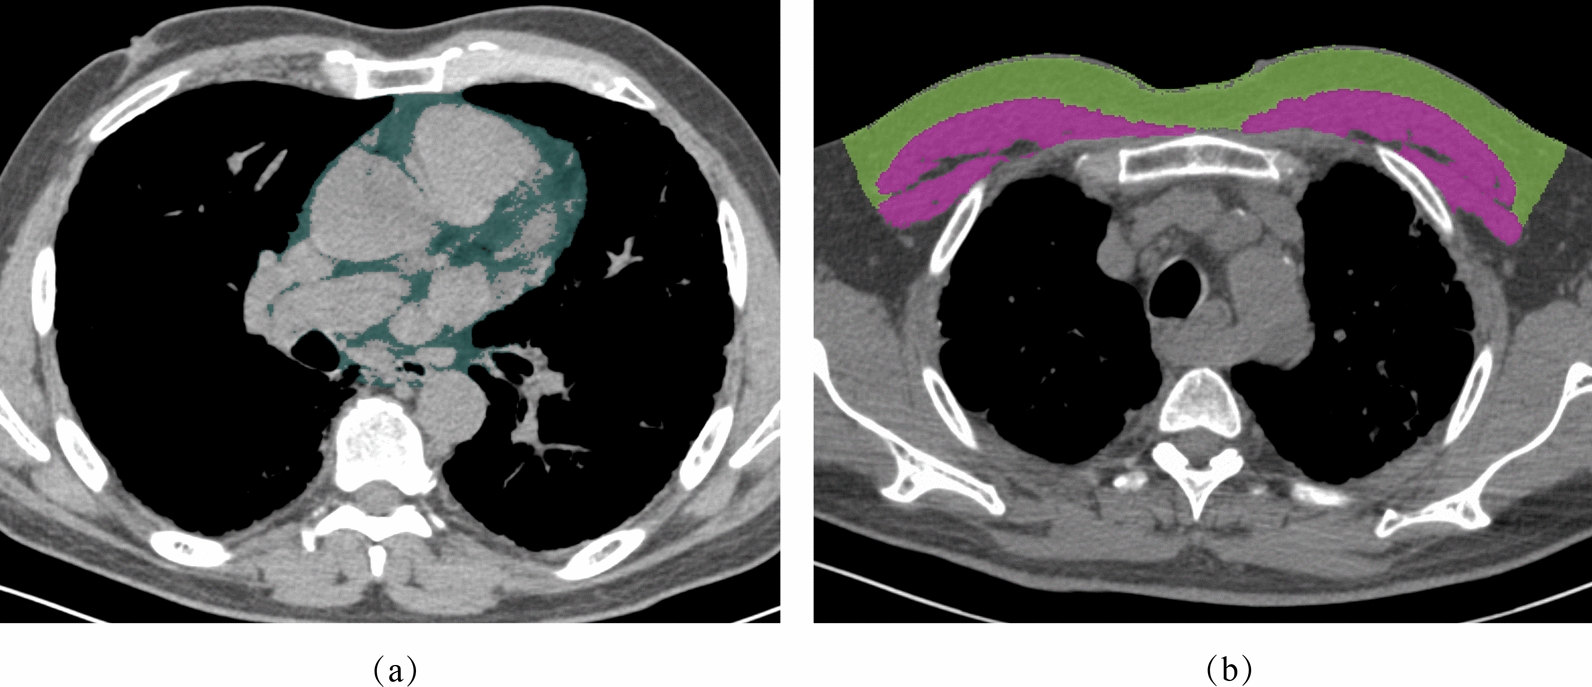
Representative CT images for the quantitation. a PAT (dark green); b pectoralis muscle (purple) and SAT of the chest wall (light green)
